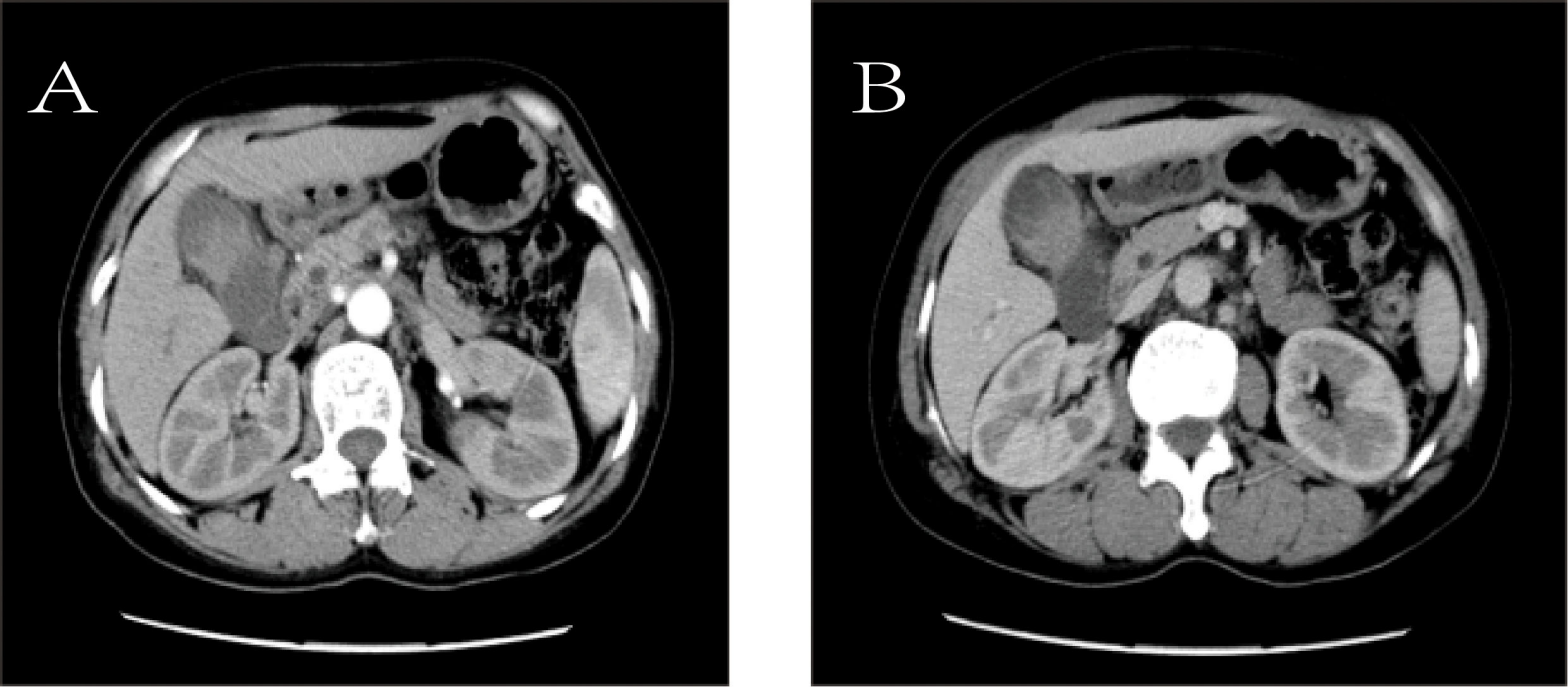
Contrast-enhanced computed tomography (CT). (A) Arterial phase image shows the mass (white arrow). (B) Venous phase image of the same mass (white arrow).
